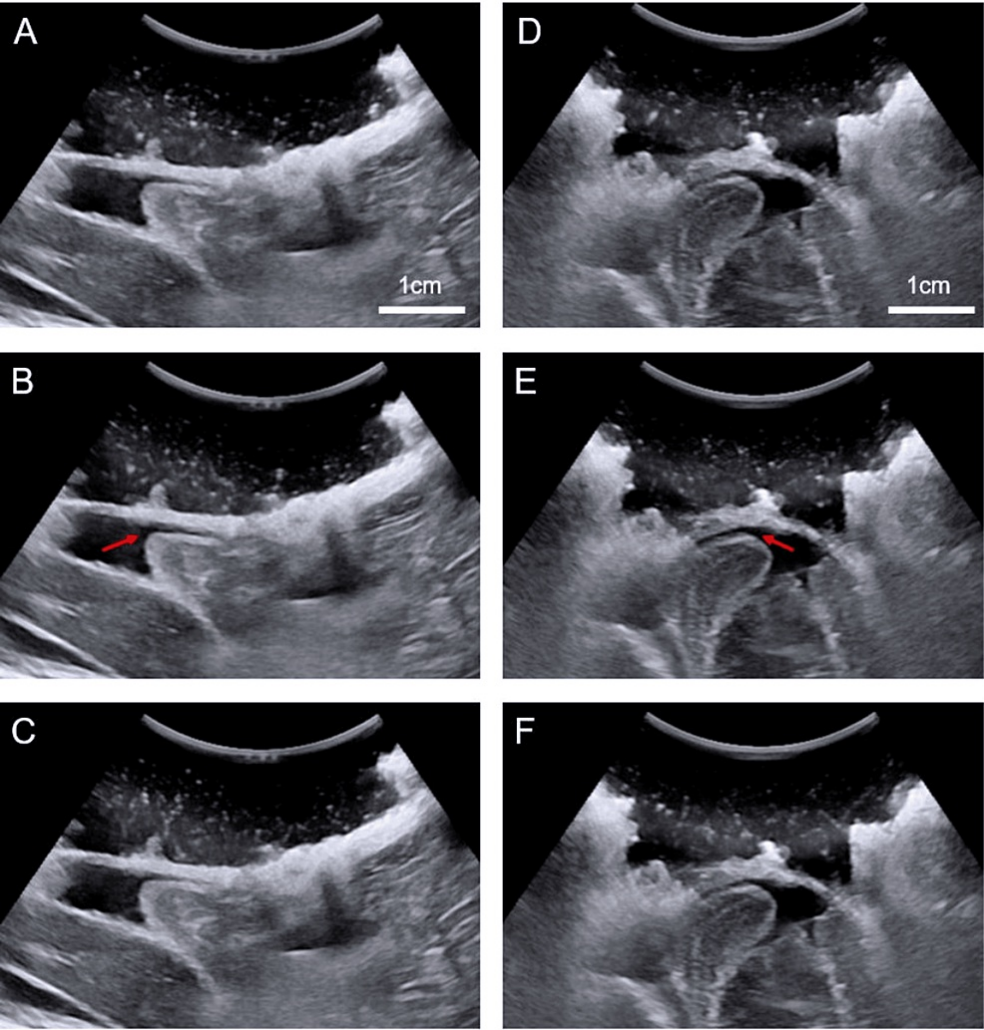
Ultrasound confirmation of cerebellar tonsil decompression with restoration of tonsillar pulsatile motion and cerebrospinal fluid (CSF) flow. A-C: After suboccipital decompression, there is restoration of tonsillar pulsatile motion and CSF flow, with each image representing three different points. The red arrow points to increased CSF flow and distance between the dura and cerebellar tonsil due to tonsillar motion. Sagittal view. D-F: Same figures as in A-C for a different patient.
